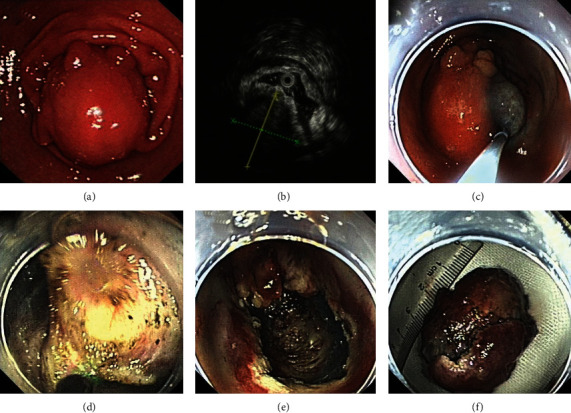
Endoscopic submucosal excavation treatment for a gastric GIST: (a) gastric GIST identified by endoscopy; (b) the same gastric GIST observed by endoscopic ultrasound; (c) marking the tumor; (d) resection of the tumor; (e) the wound after tumor removal; (f) the resected tumor. GIST: gastrointestinal stromal tumor.
